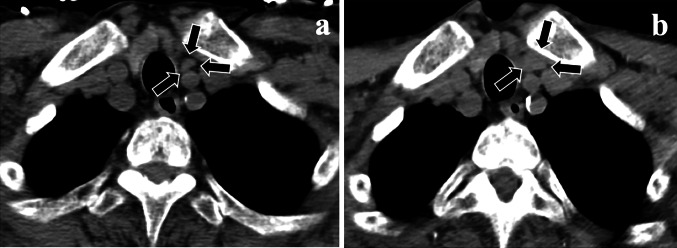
Demonstrable images of computed tomography showing the change in the size of an enlarged parathyroid gland in the neck, over 9 years. Foot note: a Arrows show an enlarged left lower parathyroid gland measuring 7.6 × 9.9 × 24.5 mm (volume: 1.30 mL) in the neck in 20XX-6. b Arrows show the same enlarged parathyroid gland measuring 11.0 × 18.6 × 28.0 mm (2.83 mL) in 20XX + 3
